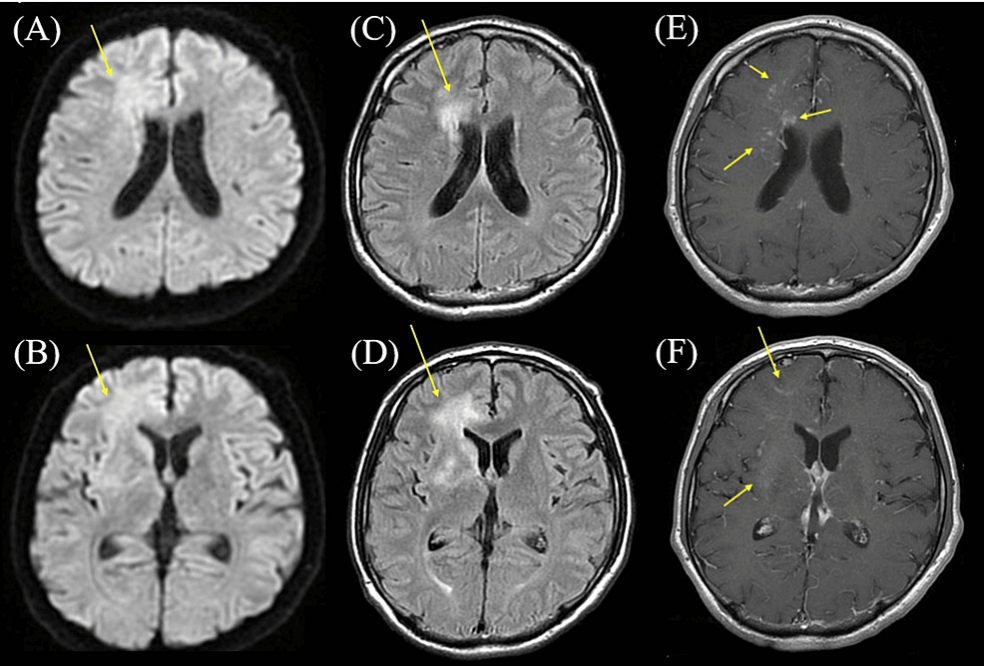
Results of the brain magnetic resonance imaging (MRI). (A, B) Diffusion-weighted brain MRI showing hyperintensity in the right frontal lobe (arrowhead). (C, D) T2-fluid attenuated inversion recovery (FLAIR) brain MRI showing hyperintensity in the right frontal lobe (arrowhead). (E, F) Gadolinium-enhanced T1-weighted brain MRI showing enhanced lesion in the area of hyperintensity by diffusion-weighted and T2-FLAIR MRI.
